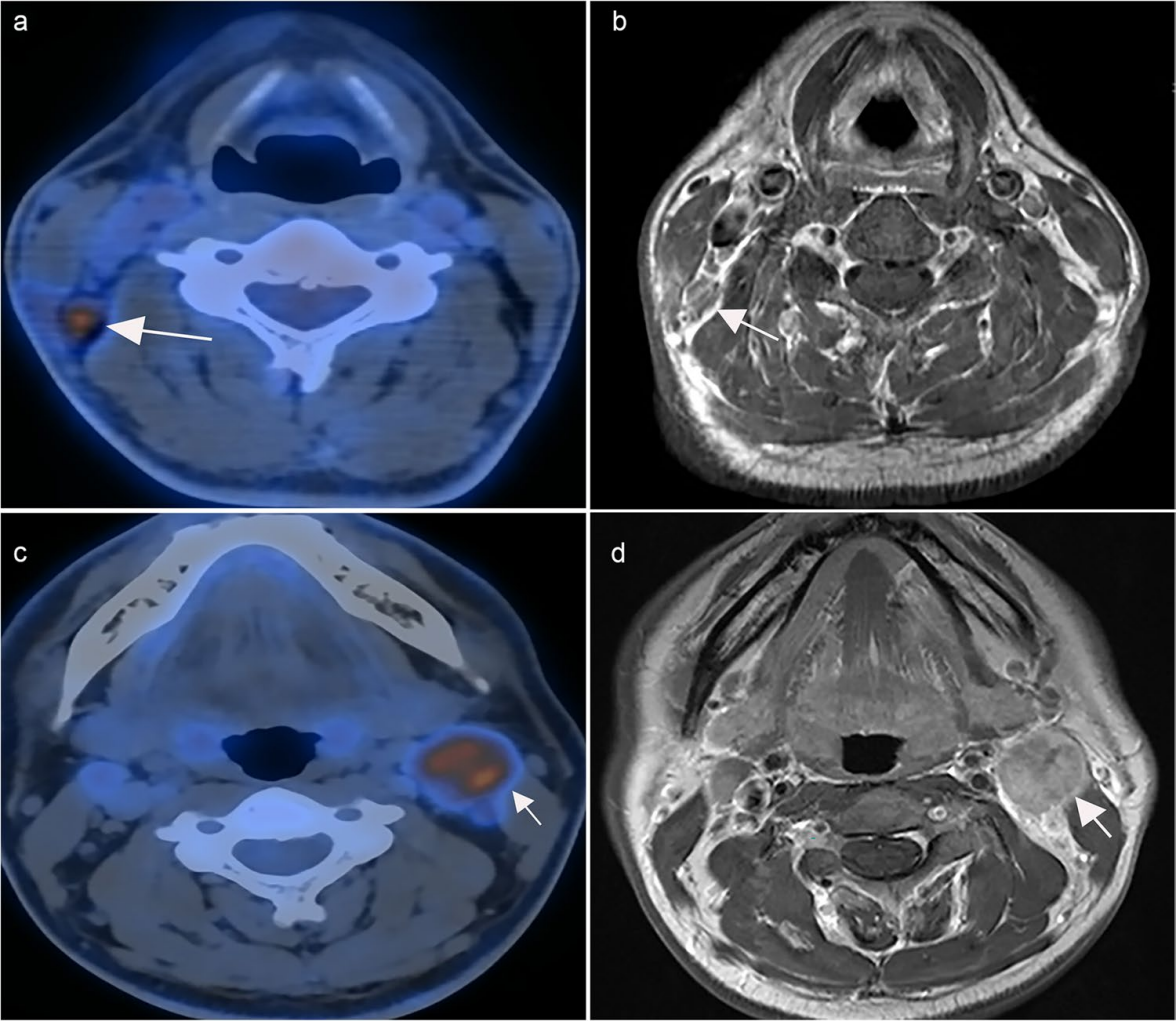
Cervical lymph nodes in PET/CT (left) and contrast-enhanced T1-weighted MRI (right), a PET/CT correctly diagnosed positive lymph nodes, while MRI diagnosed negative lymph nodes by mistake (b); both PET/CT (c) and MRI (d) correctly detected metastatic lymph nodes. Abbreviations: MRI, magnetic resonance imaging; PET/CT, [18F]-fluorodeoxyglucose positron emission tomography with computed tomography
